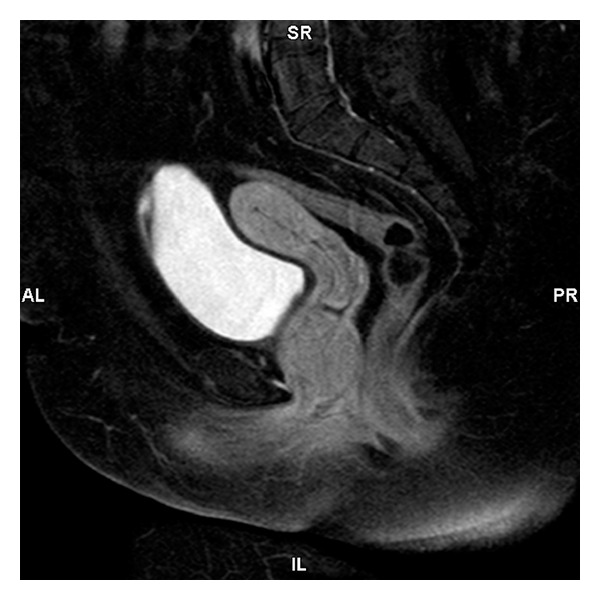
T2-weighted MRI of the pelvic characterized a 4.6 × 4.2 × 4.0 centimeter mass located within the anterior vaginal wall with mild homogeneous enhancement.
